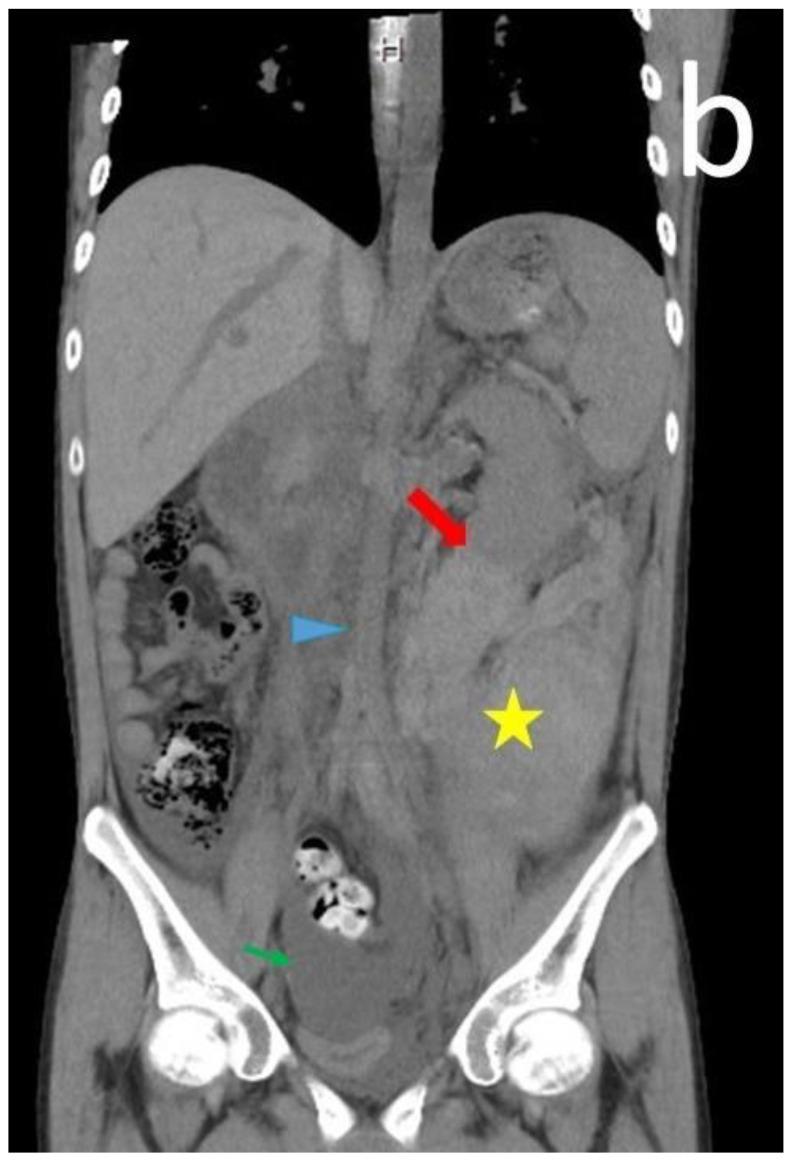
This is a figure showing the CT of the abdomen at the emergency department. Axial view (a) and coronal view (b). There was a large retroperitoneal hematoma in the left retroperitoneal space (yellow asterisk) and suspected active bleeding in the left kidney lower portion (red thick arrow). Mild ascites (green thin arrow) and decreased volume with a small caliber of the aorta and inferior vena cava (blue arrowhead) were noted.
